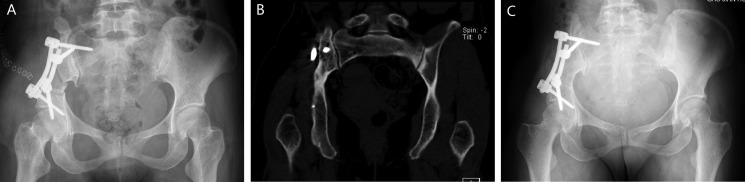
A 44-year-old woman (case 6) with diagnosis of pelvis osteoclastoma affecting zone I (A) AP radiograph of the pelvis after bone tumor resection and reconstruction with an autograft bone in the pelvis stabilized with the implantation of screw-rod system. (B) Coronal CT image showing the bone union 17.5 months post-operatively. (C) AP radiograph of the pelvis shows no evidence of tumor recurrence or implant loosening 36 months post-operatively.
